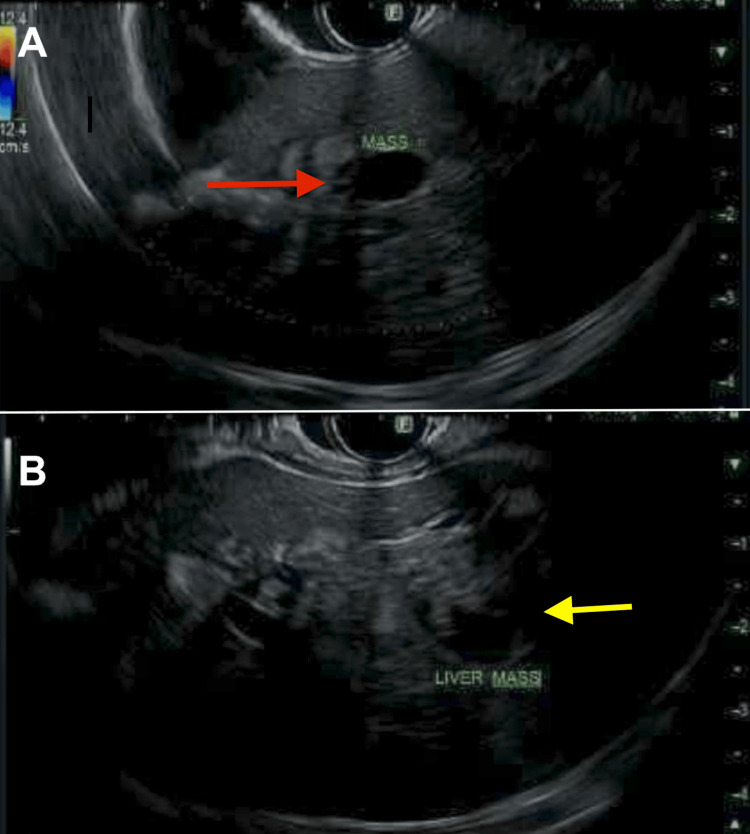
EUS images (A, B) Left hepatic hyperechoic mass seen on EUS, measuring 40 x 30 mm in maximal cross-sectional diameter (arrows). EUS: endoscopic ultrasound.
